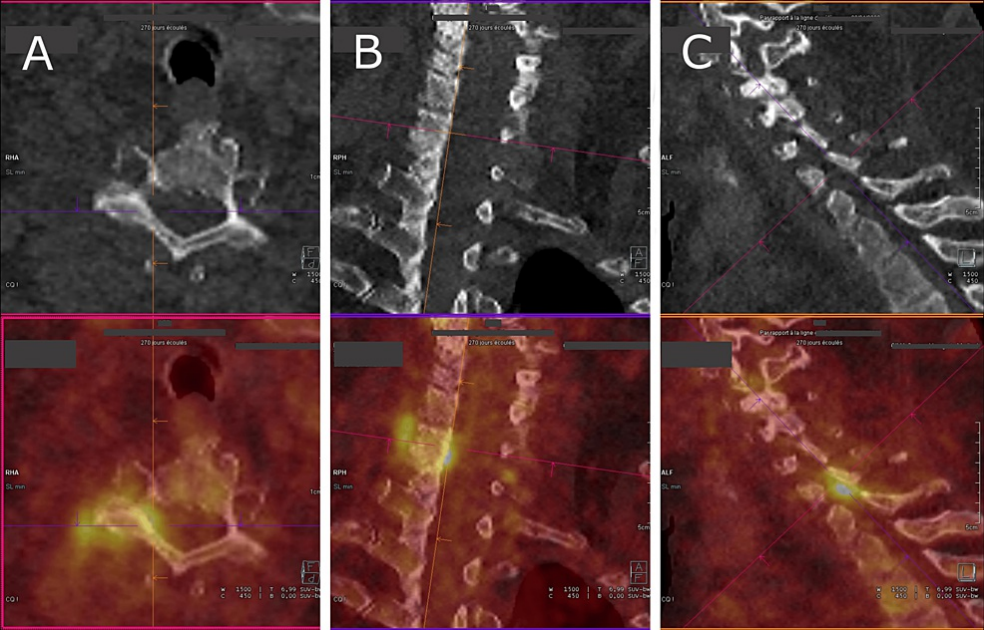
PET scan showing hypermetabolic activity in the right inter-apophyseal joint C6-C7 (SUV=5) A: axial view; B: coronal view; C: sagittal view PET, positron emission tomography
